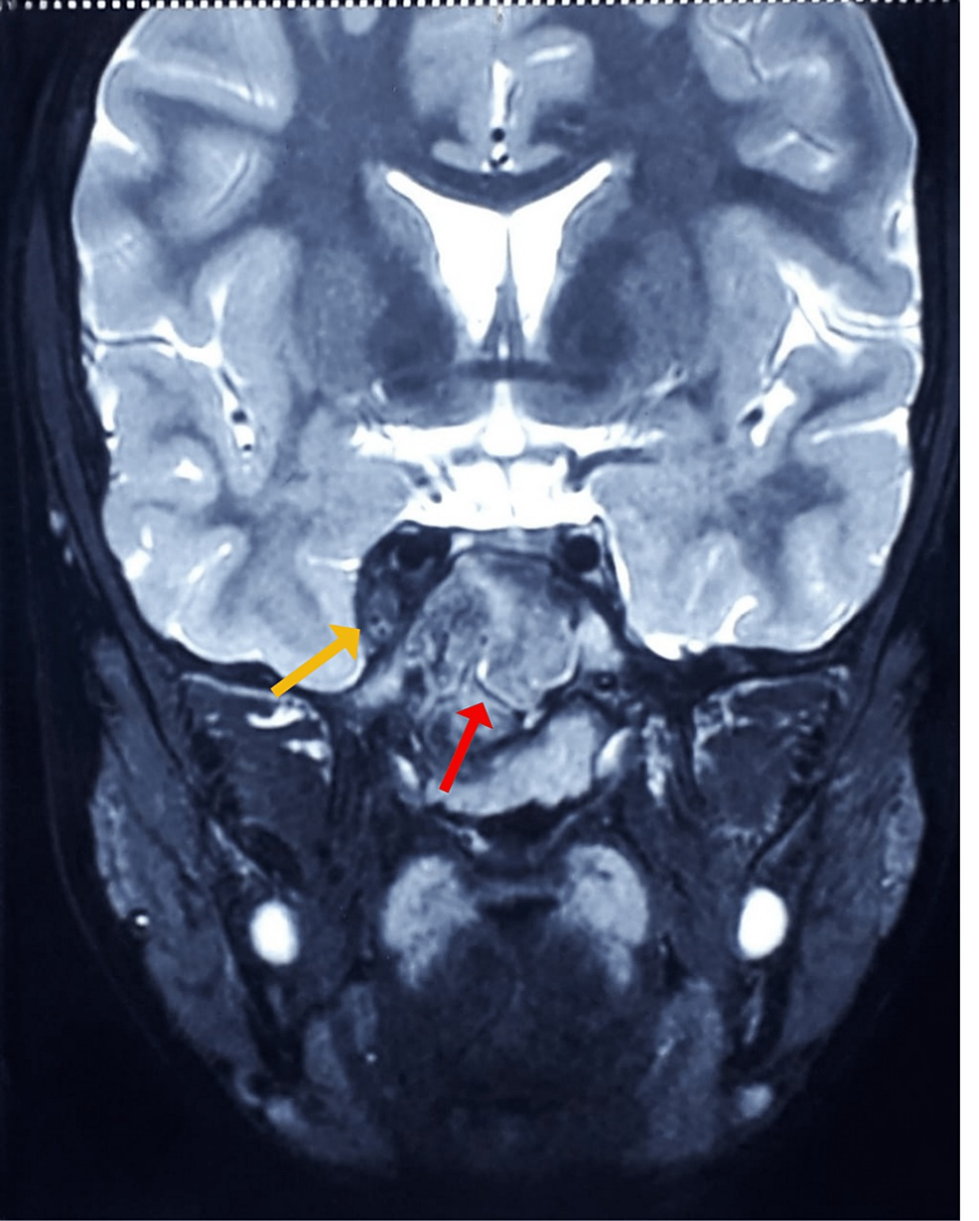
T2 weighted image showing an iso-hypointense mass lesion involving sphenoid sinuses (red arrow) and invading right cavernous sinus (yellow arrow) in magnetic resonance imaging of the brain, paranasal sinuses, and orbits
